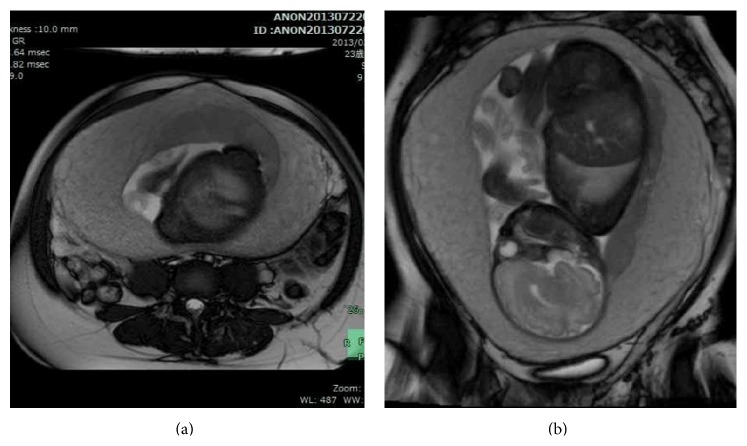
T2-weighted MRI showed a greatly enlarged uterus and diffuse myometrial thickening with a visible junctional zone between the endometrium and large high-intensity myometrium at 30 weeks of gestation ((a) transverse plane and (b) coronal plane).
